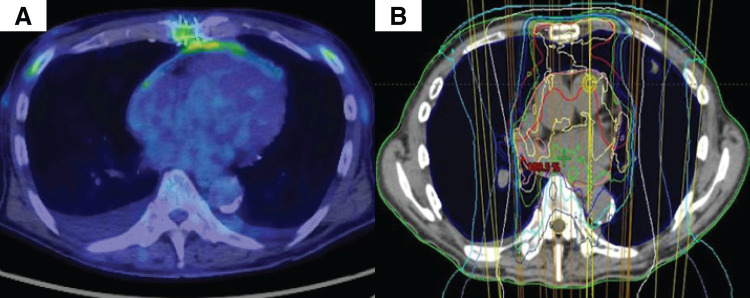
PET-CT at POD 46 shows no abnormal accumulation of fluorodeoxyglucose (A). Images showing postoperative radiation therapy planning (B).
